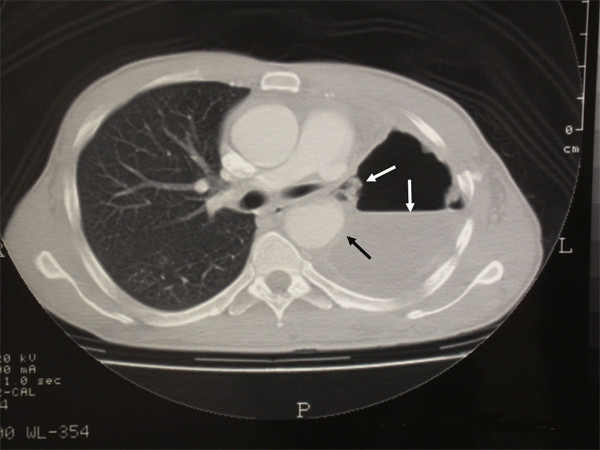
Axial CT scan (window setting) shows air-liquid level and bronchopleural fistula (white arrows). The aortic prosthesis is covered by purulent pleural fluid (black arrow).
